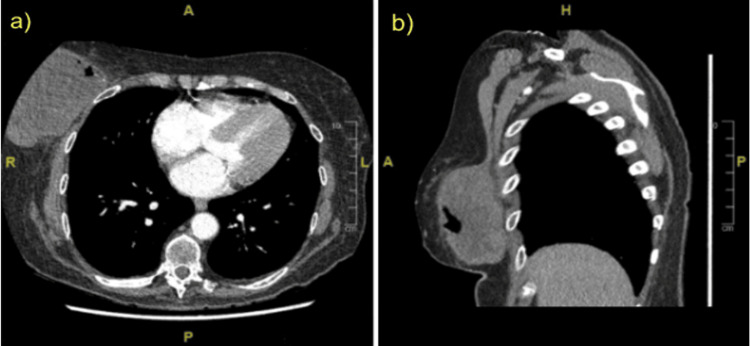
CT chest showing (a) axial and (b) sagittal views of the right breast lesion on initial presentation to the hospital, demonstrating cystic components within the anterior aspect of the mass CT: computed tomography
